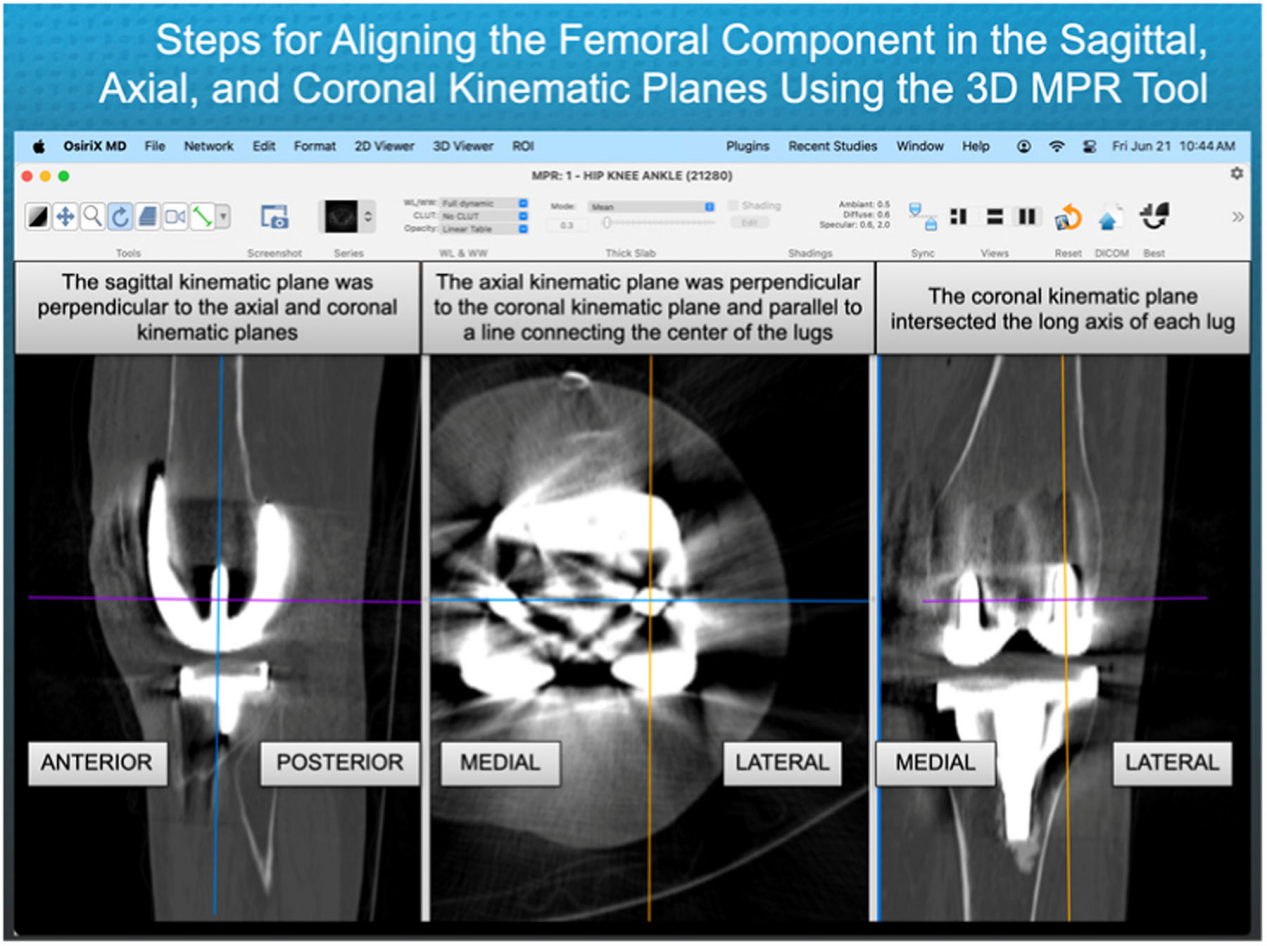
The image displays the menu bar containing the software settings for viewing the CT scan of the KA TKA. It demonstrates the steps for using the lugs of the femoral component to orient the knee in the sagittal, axial and coronal kinematic axis reference planes using the 3D multiplane (MPR) viewer. CT, computed tomography; KA TKA, kinematically aligned total knee arthroplasty; MPR, Multiplane Reconstruction Tool.
